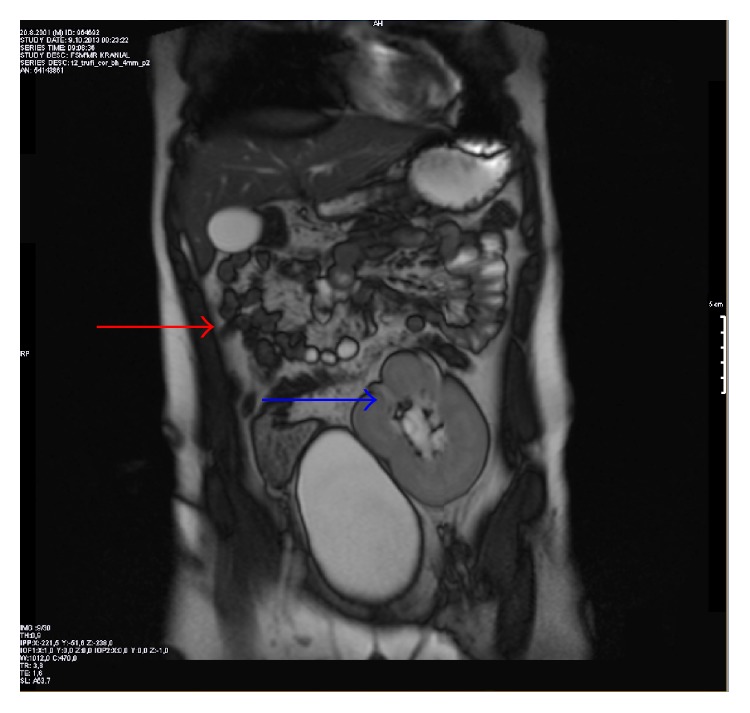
Magnetic resonance imaging of abdomen on the coronal plane. There were right renal agenesis (red arrow) and left ectopic pelvic kidney over the urinary bladder (blue arrow).
